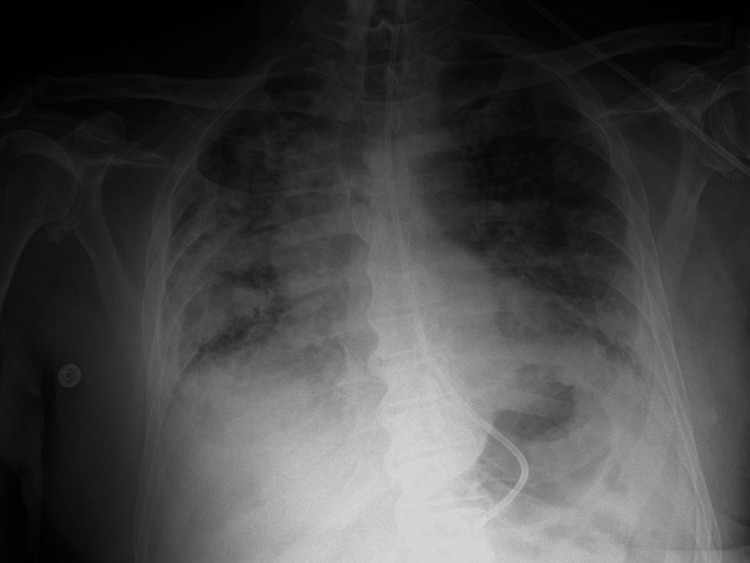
Chest radiograph demonstrating bilateral pulmonary opacities consistent with pulmonary haemorrhage, more pronounced in the right side. This image shows diffuse patchy opacities, greatest in the right mid-to-lower zones, consistent with vasculitic pulmonary involvement in eosinophilic granulomatosis with polyangiitis.
